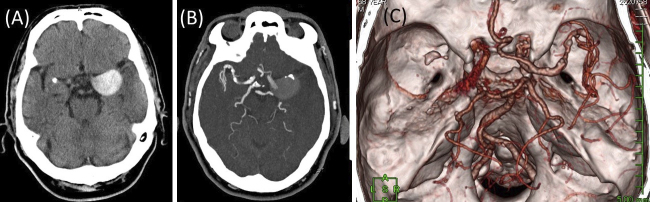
Computed tomography (CT) on admission revealed a high-density, round mass in the left middle cerebral artery (MCA), which was suspected to be an unruptured giant cerebral aneurysm (A). CT angiography revealed MCA occlusion and an aneurysm in the proximal part of the left MCA with poor opacification, suggesting partial thrombosis (B, C).
